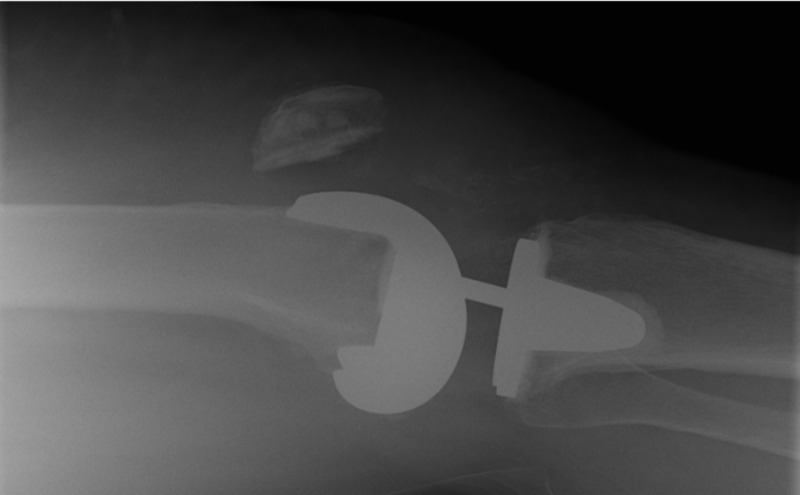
Preoperative lateral x-ray showing a patella alta
